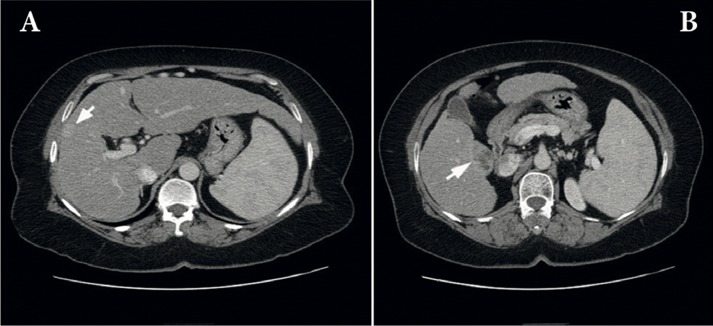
Dynamic Computed Tomography (CT) Before Liver Transplantation A) A 1.5-cm-size focal hepatic lesion with typical vascular pattern of hepatocellular carcinoma (HCC) in segment 8; B) A 3-cm-size focal hepatic lesion with atypical vascular pattern of HCC in segment
